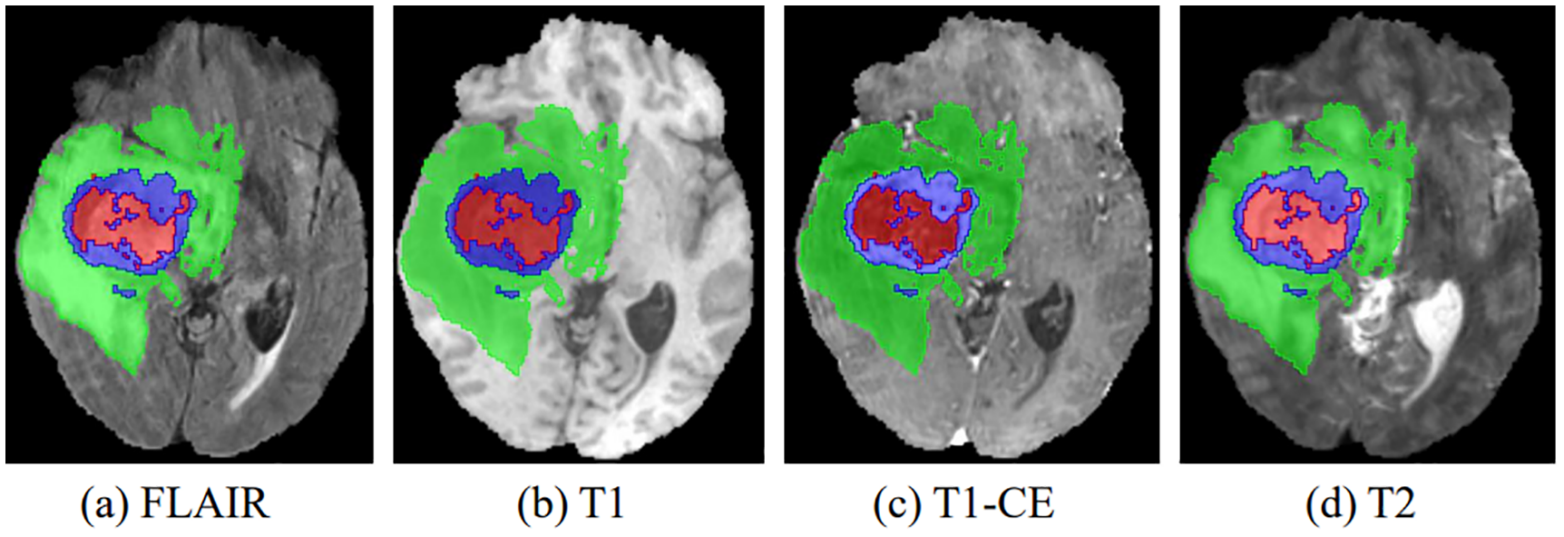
The four multimodal MR images with glioma tumors are: (A) FLAIR, (B) T1, (C) T1-CE, and (D) T2. Different colors are used to differentiate tumor subregions: red for necrotic and non-enhanced tumor (NCR/NET), green for peritumoral edema (ED), and blue for enhanced tumor (ET).
